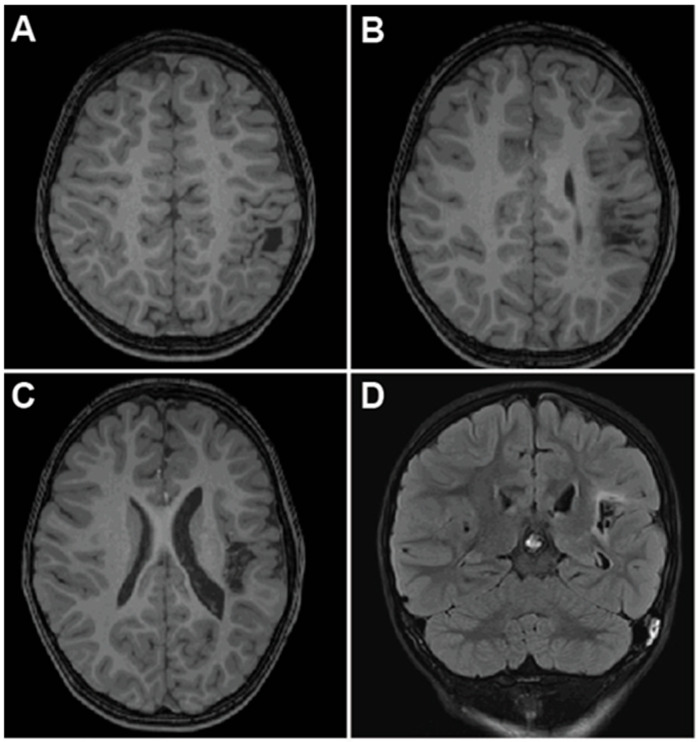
Preoperative axial T1-weighted (A–C) and coronal FLAIR (D) MRI for Case 3 demonstrating region of encephalomalacia spanning posterior insula through to the left parietal lobe.
